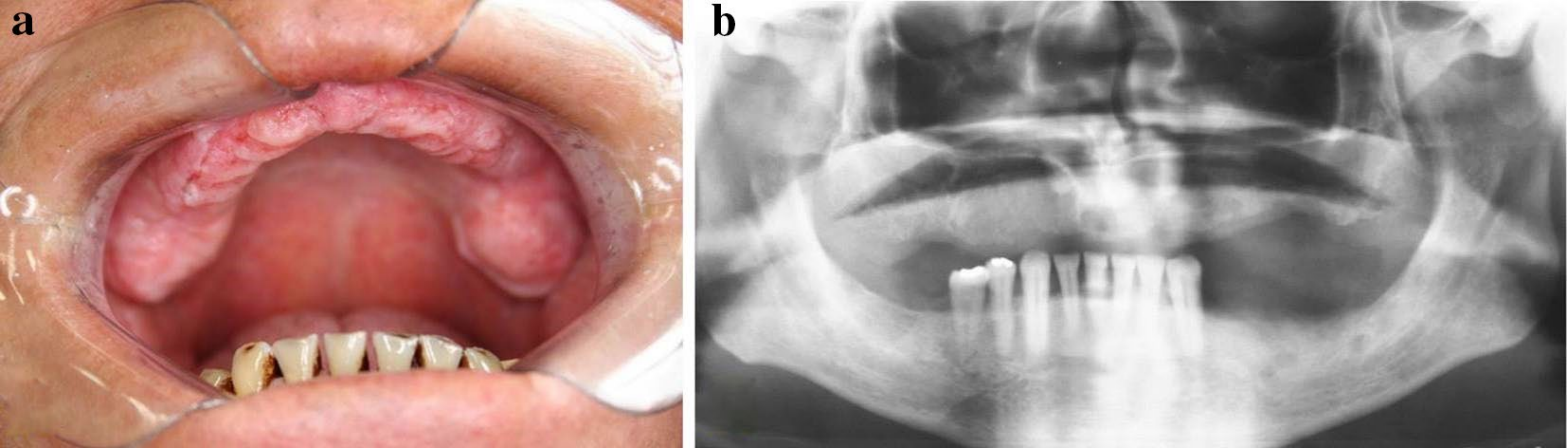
Intraoral (a) view and panoramic radiograph (b) showed no bone disease (8 years later during follow-up)
